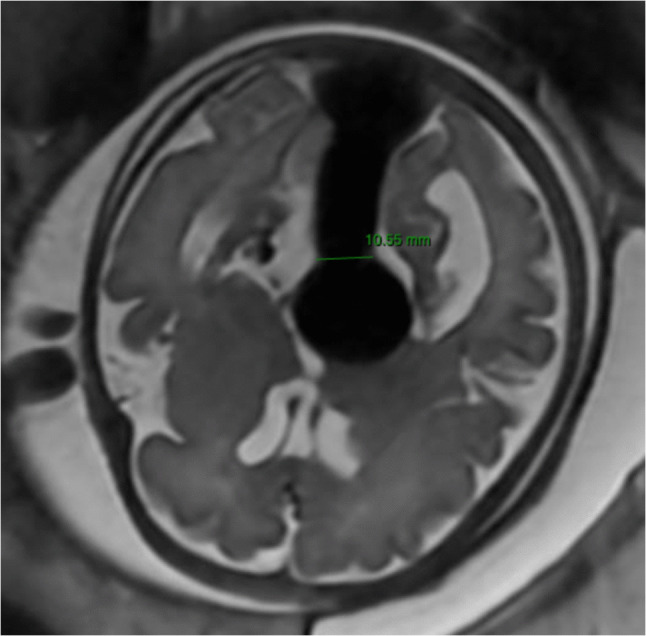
Fetal MRI of the clinical case showing measurement of the falcine sinus at its narrowest point, measuring 10.5 mm
